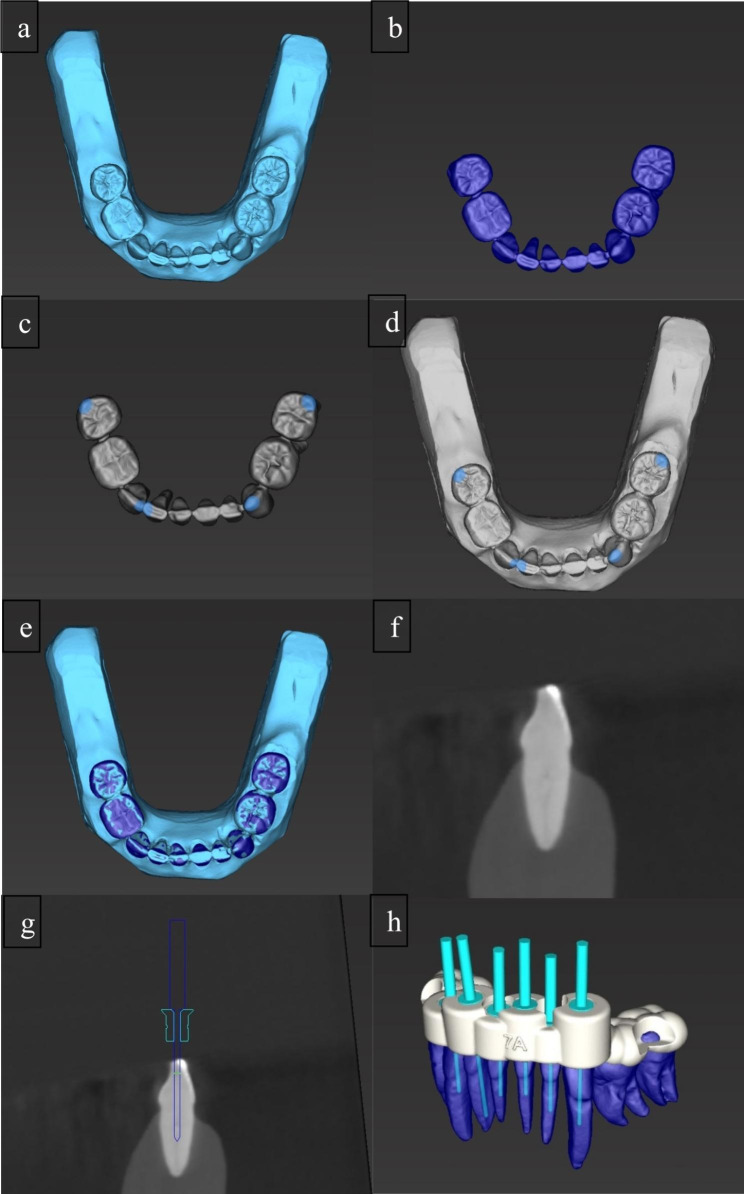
The intraoral scan (a) and the CBCT image (b) were matched in the planning software (c to e). Virtual drills were placed to the root canal orifices to provide a straight-line access (f and g) and a template was designed (h)
